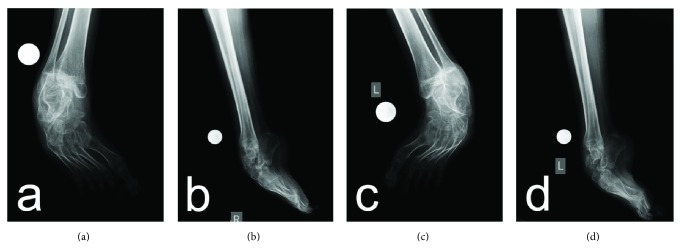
Preoperative anteroposterior (a, c) and lateral (b, d) radiographs of the patients with hereditary motor and sensory neuropathy type I.
